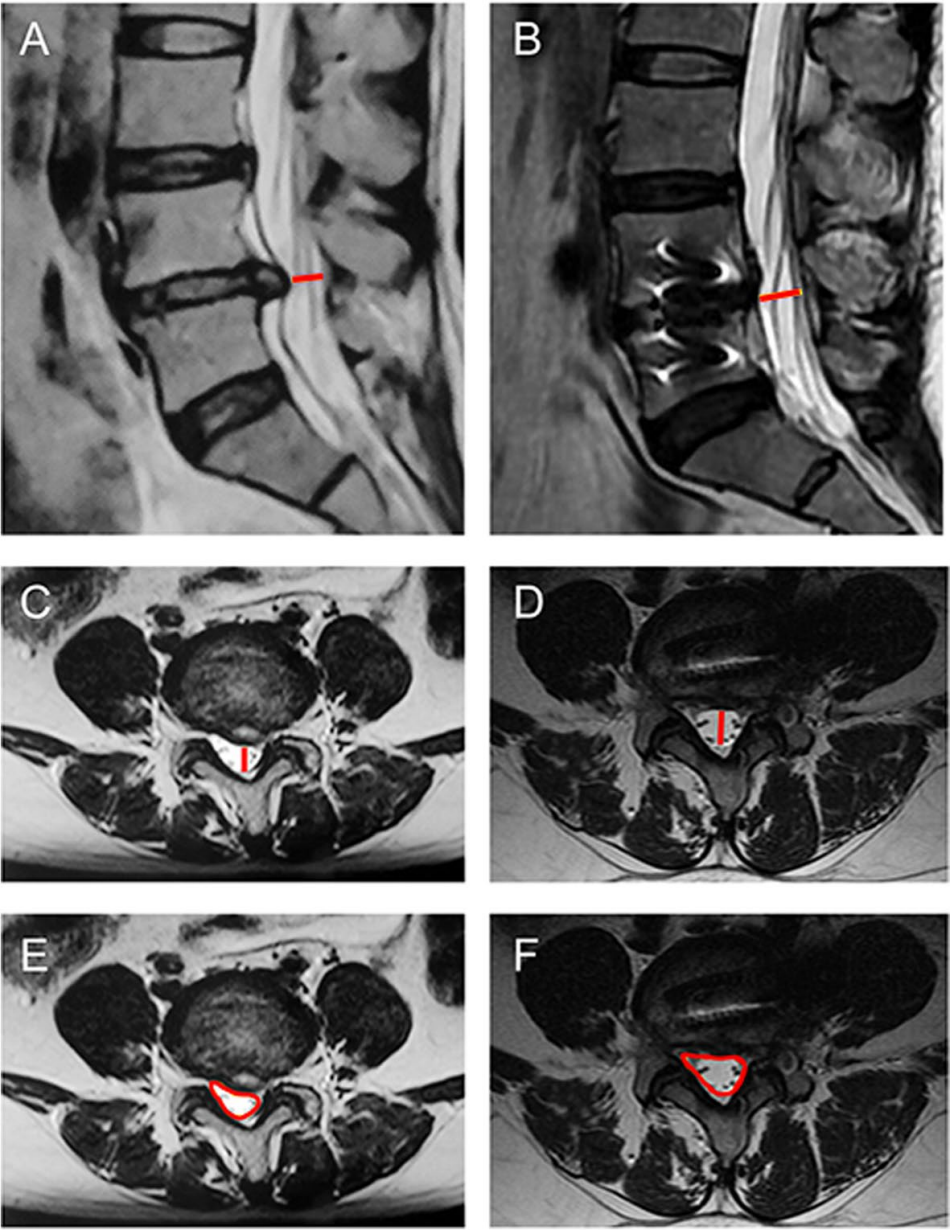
Measurement of MRI-related indexes. A, B SSCD was measured on preoperative (A) and postoperative (B) lumbar MRI sagittal images: anterior and posterior length of the spinal canal at the central level of the intervertebral space. C, D ASCD was measured on preoperative (C) and postoperative (D) lumbar MRI axial images: the length of the spinal canal at the anterior and posterior levels. E, F SC-CSA was measured on preoperative (E) and postoperative (F) lumbar MRI axial images
